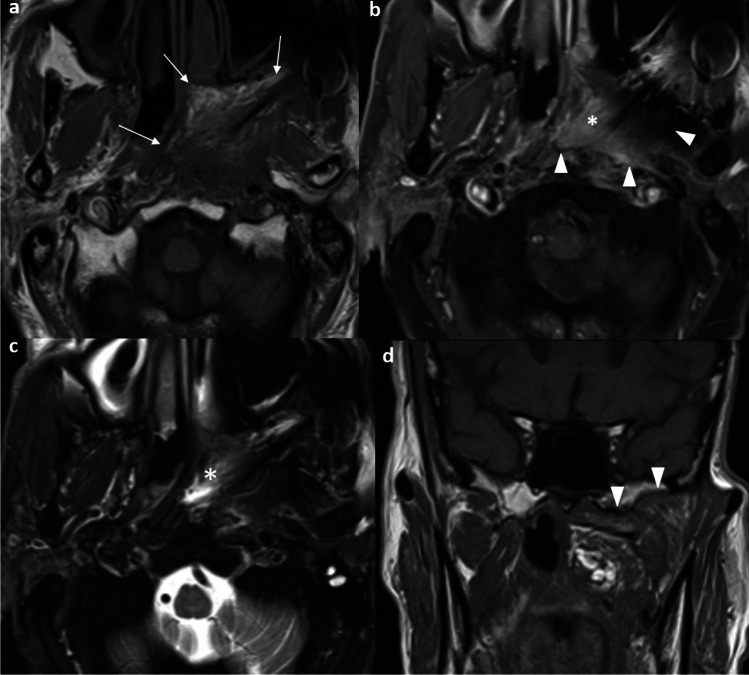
Normal expected post-operative appearance of open nasopharyngectomy in a 58-year-old man with NPC (clinical stage T4N3M0) completed chemo-irradiation in 2017. Two years after, he had a left retropharyngeal node recurrence on surveillance MRI (not shown) and subsequently an open nasopharyngectomy via maxillary swing approach and a flap reconstruction using temporalis muscle. Axial T1-weighted pre-contrast image without fat suppression one-year after the operation shows no recurrence, demonstrating normal fat striation of the temporalis muscle flap without soft tissue replacement (arrows) (a). Axial T1-weighted post-contrast image with fat-suppression shows non-mass like enhancement in the medial aspect of the flap (asterisk). The recipient bed of the flap shows a straight margin without discrete nodular enhancement (arrowheads) (b). Axial T2-weighted image with fat suppression shows mild non mass-like hyperintense signal (asterisk) (c). Coronal T1-weighted image without fat-suppression shows the smooth transition in the surgical bed and straight superior margin of the flap with respect to the sphenoid bone (arrowheads) (d)
